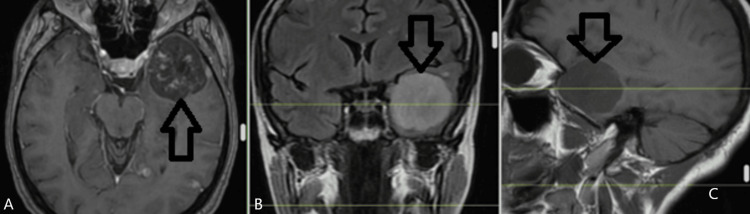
MRI shows a left temporal lobe lesion A: axial plane; B: coronal plane; C: sagittal plane
